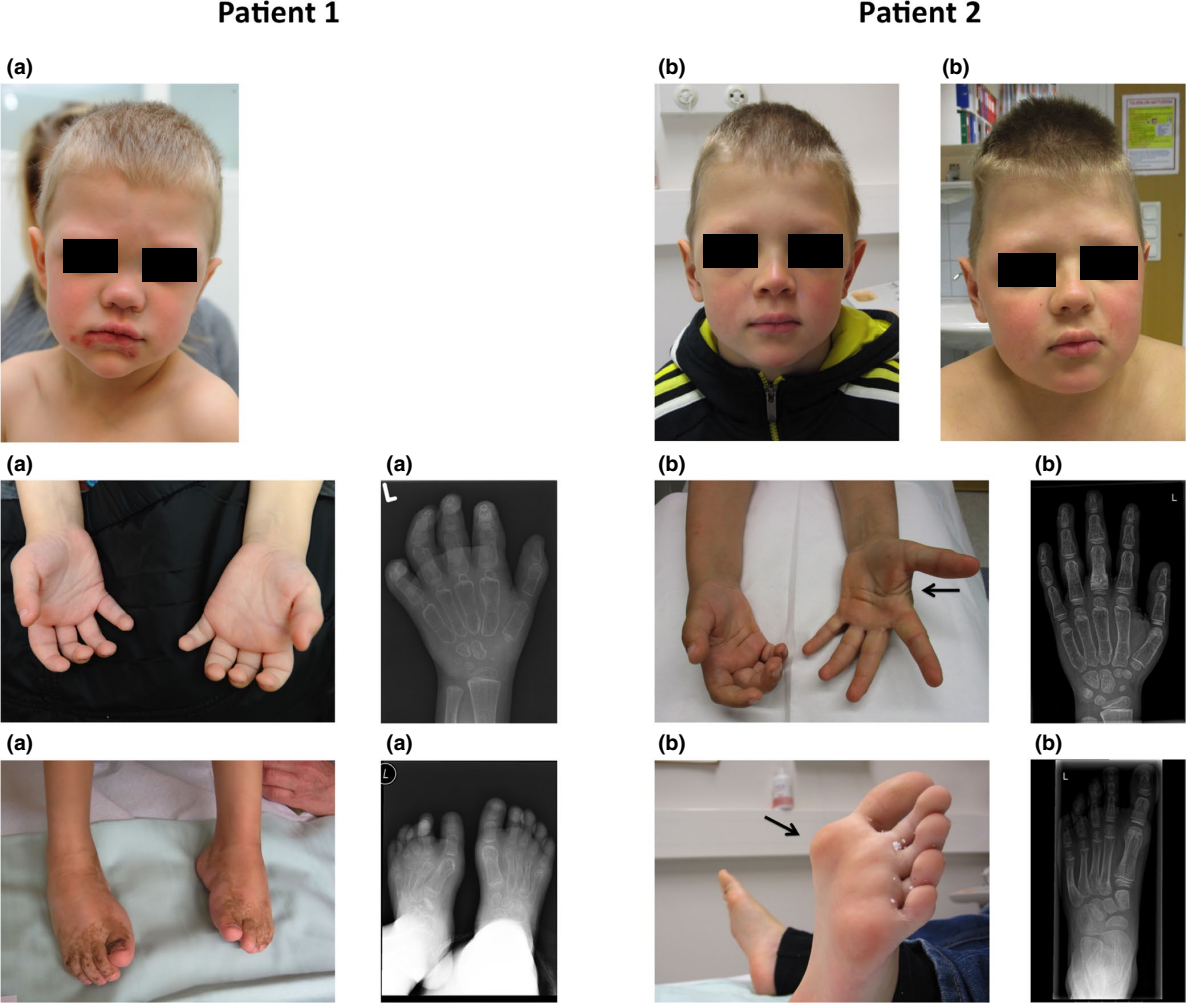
Patient 1 (a) at the age of 3 years and his older brother (Patient 2) (b) at the ages of 8 and 11 years both showing minor facial dysmorphic features: bulbous nose and frontal bossing. The younger patient (a) had joint contractures in both hands and feet. The older one (b) suffered from painful subcutaneous nodules in the plantar area and tendon restrictions in the hands. Radiographs demonstrate bone loss, wide metacarpals, and metatarsal bones
